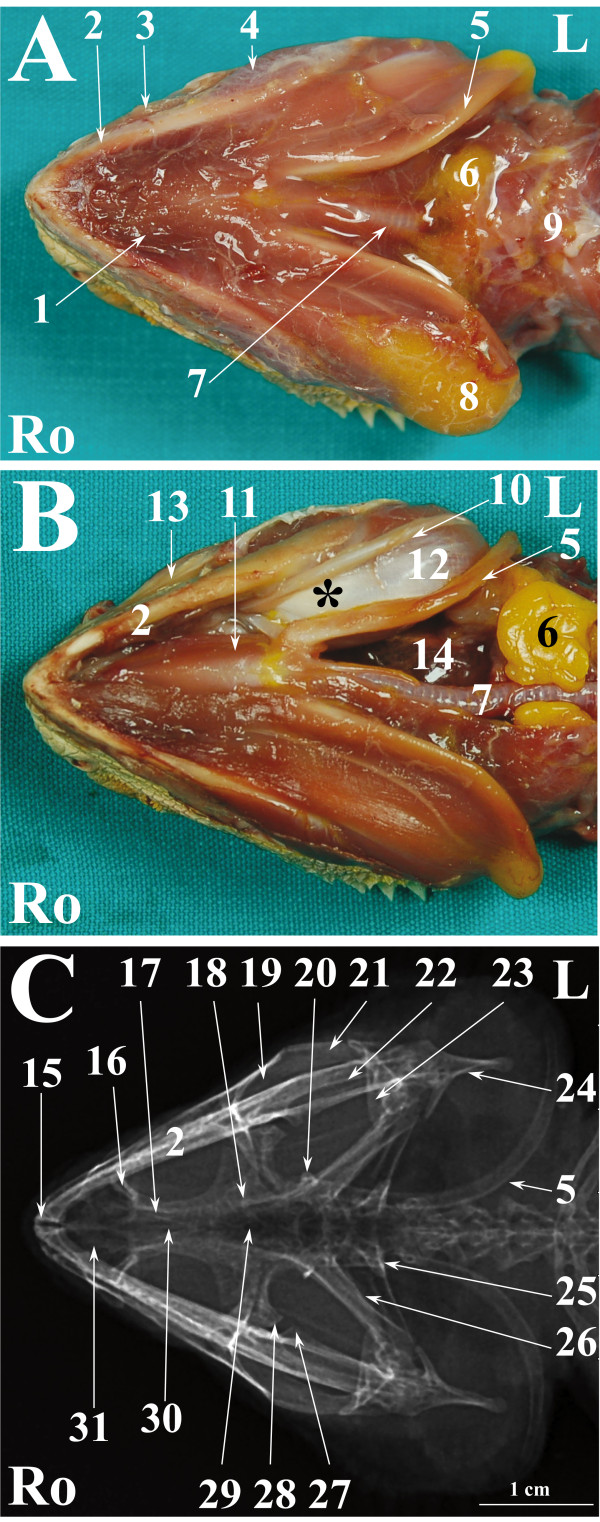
Normal radiographic anatomy of the head of the bearded dragon in VD projection.A. Ventral view in a superficial plane of stratigraphic dissection (only skin was removed) of the head of a bearded dragon. B. Ventral view of stratigraphic dissection after removal of Musculus constrictor colli and Musculus intermandibularis (lower part of the image) and a deeper plane of dissection (upper part of the image) of the head of a bearded dragon (deeper plane of dissection is labelled with * on the image). C. VD radiographic projection of the head of a bearded dragon. Ro is rostral, L is left.1. Musculus intermandibularis; 2. Dentary bone; 3. Supralabial gland; 4. Musculus adductor mandibulae externus supeficialis; 5. Ceratobranchial 1 process of hyobranchial skeleton; 6. Fat body; 7. Trachea; 8. Fat body; 9. Musculus constrictor colli; 10. Ceratohyal process of hyobranchial skeleton; 11. Basihyal bone; 12. Musculus pterygoideus typicus; 13. Maxillary bone; 14. Oesophagus; 15. Premaxillary bone; 16. Prefrontal bone; 17. Palatine bone; 18. Frontal bone; 19. Jugal bone; 20. Epipterygoid bone; 21; Squamosal bone; 22. Angular bone + Surangular bone; 23. Quadrate bone; 24. Articular bone; 25. Paraoccipital process of otooccipital bone; 26. Pterygoid bone; 27. Coronoid bone; 28. Ectopterygoid bone; 30. Interpterygoid vacuiti; 31. Vomer bone.
